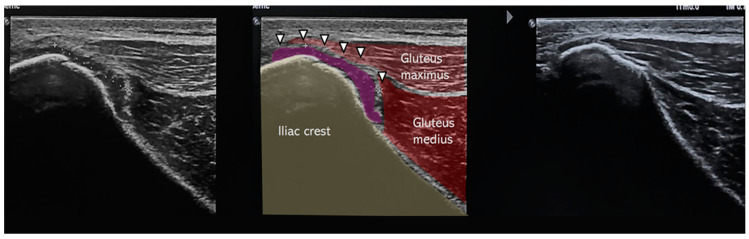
Sagittal ultrasound scans of posterior iliac crest in Case 1: (left) injured side, (middle) annotated scan highlighting periosteal detachment (arrowhead), and (right) uninjured side for comparison.
